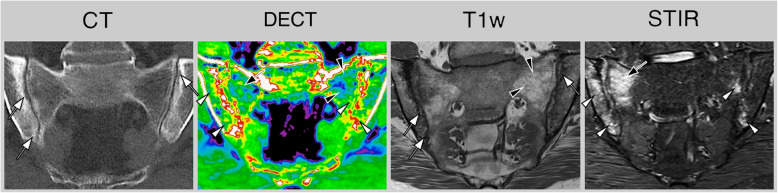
Dual-energy computed tomography (DECT) of a patient with axial spondyloarthritis. Computed tomography (CT) and T1-weighted imaging reveal evidence of erosion (white arrows) and sclerosis. DECT shows increased density in regions characterized by bone marrow edema (white arrowheads) as well as decreased density in adipose tissue lesions (black arrowheads). Additionally, there is a notable mixed density observed in a specific region exhibiting bone marrow edema within a fat lesion (indicated by a black arrow)
